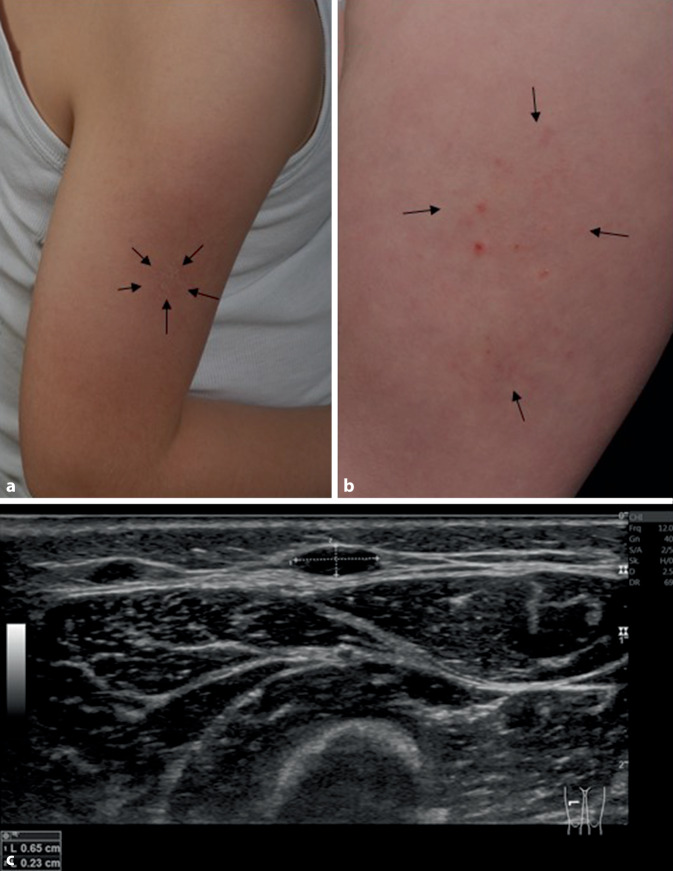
a Persistierende Ekzemreaktion (*Pfeile*) der Haut bei einem 7‑jährigen Jungen (Patient 6) nach quadrivalenter Masern-Mumps-Röteln-Impfung in den rechten Oberarm. b Persistierende Ekzemreaktion (*Pfeile*) bei einem 6‑jährigen Jungen nach hexavalenter (Diphtherie, Tetanus, Pertussis, Polio, Haemophilus influenzae B, Hepatitis B) Impfung in den linken Oberschenkel. (Patient 8) c Sonographie eines Impfgranuloms des ventrolateralen Oberschenkels rechts bei einem 2‑jährigen Jungen (Patient 4)
